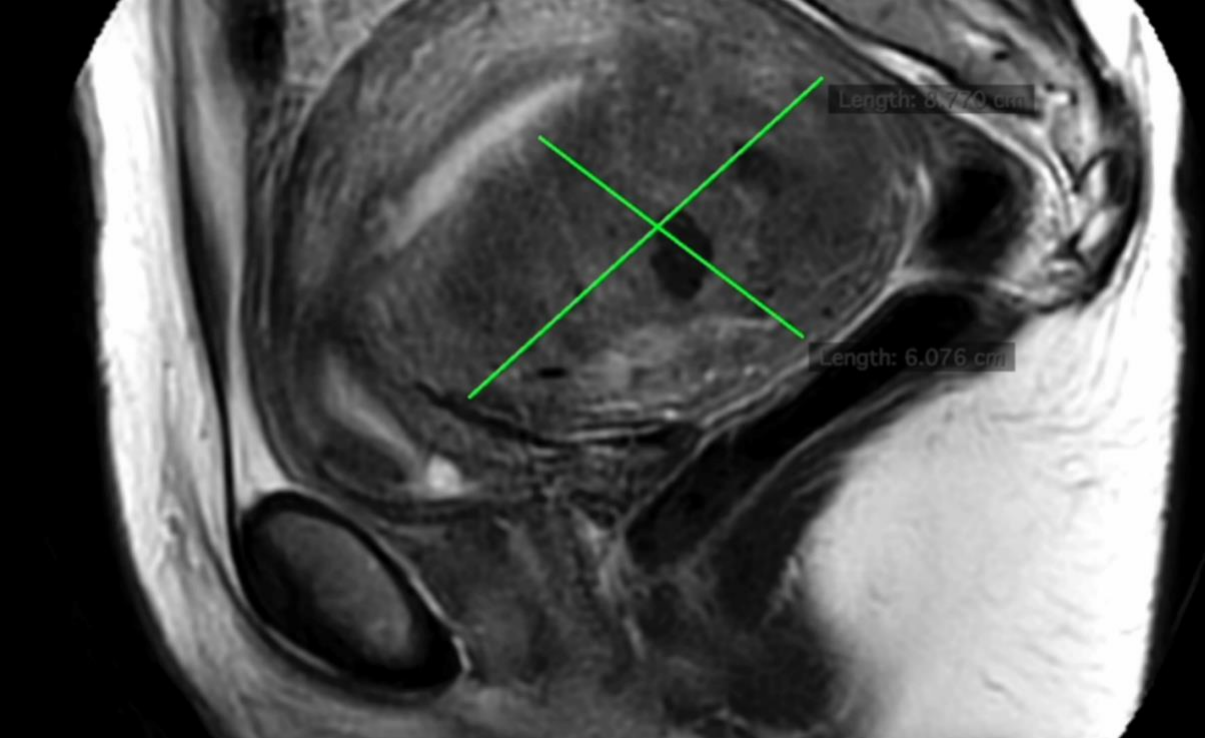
MRI image showing a posterior wall diffuse adenomyosis (8.7 x 6.0 cm) with a thickened junctional zone.
